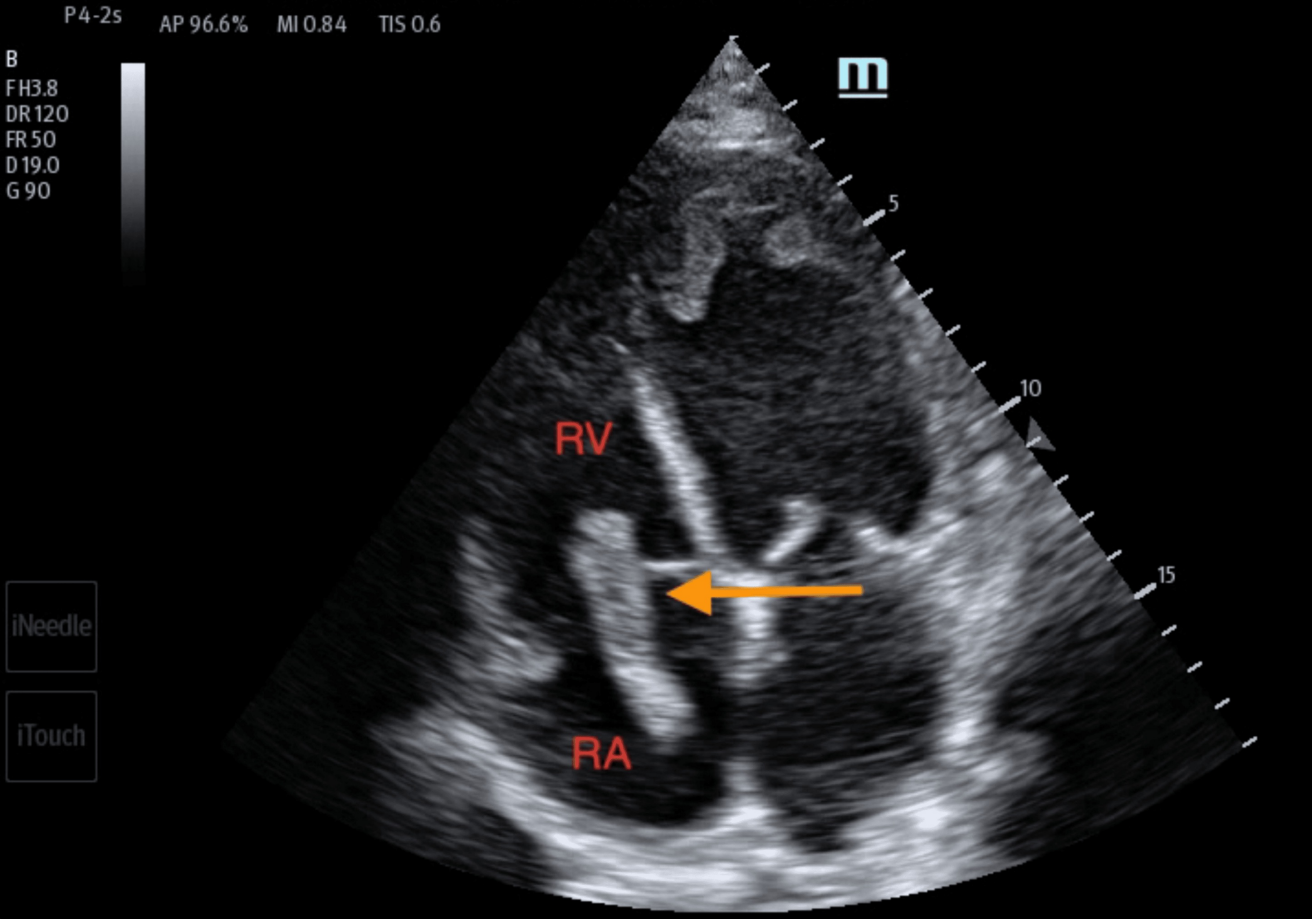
Transthoracic echocardiogram imaging of the apical four-chamber view showing long, serpiginous, and mobile thrombus (orange arrow) in the right heart chambers. RA: right atrium; RV: right ventricle
